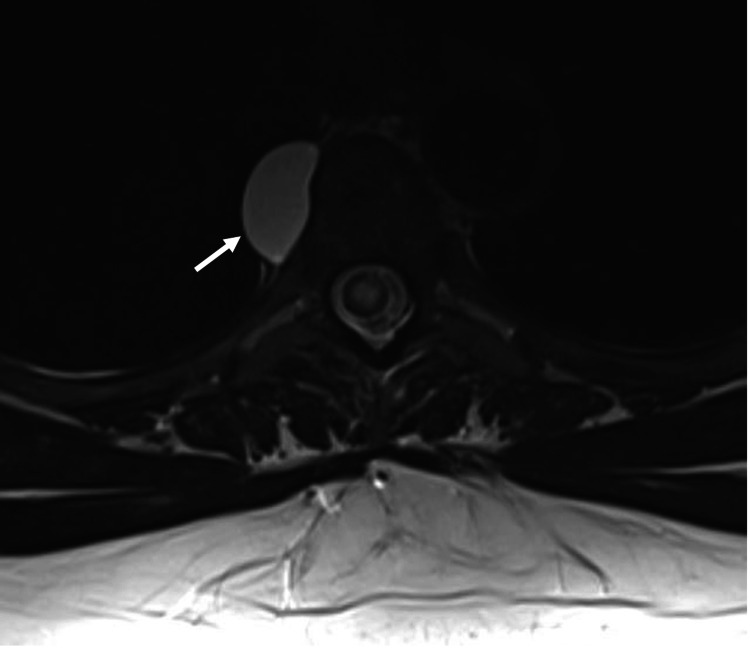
Axial MRI T2 sequence demonstrating the right-sided T5 paraspinal cystic lesion.
